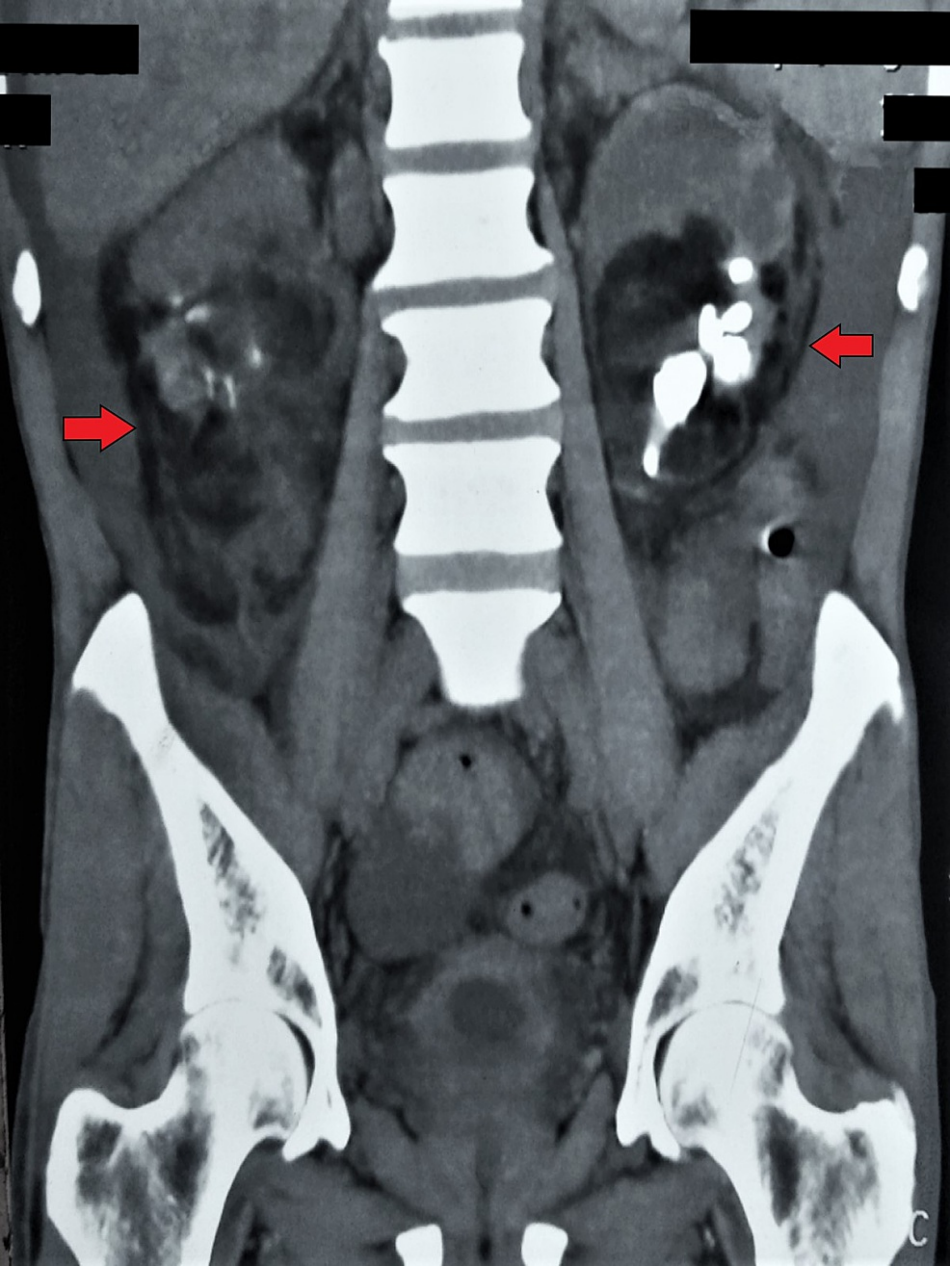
Reconstructed coronal section of the non-contrast computed tomographic scan of abdomen demonstrating bilateral renal replacement lipomatosis (red arrow) and multiple renal calculi.
